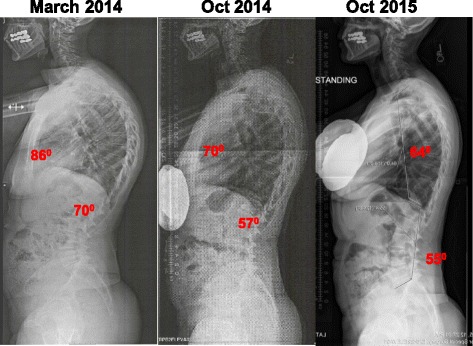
Patient’s before and after x-ras. X-ray on left, taken in March 2014 before beginning therapy showsthoracic kyphosis measuring 86° and lumbar lordosis 70°. X-ray in middle, taken in October 2014, shows patient’s results after 6 months of intensive therapy, with thoracic kyphosis measuring 70° and lumbar lordosis 57°. X-ray on right, taken in October 2015, 1 year after study completed, shows an additional significant decrease in the SK angle with thoracic kyphosis measuring 64° and lumbar lordosis 55°
